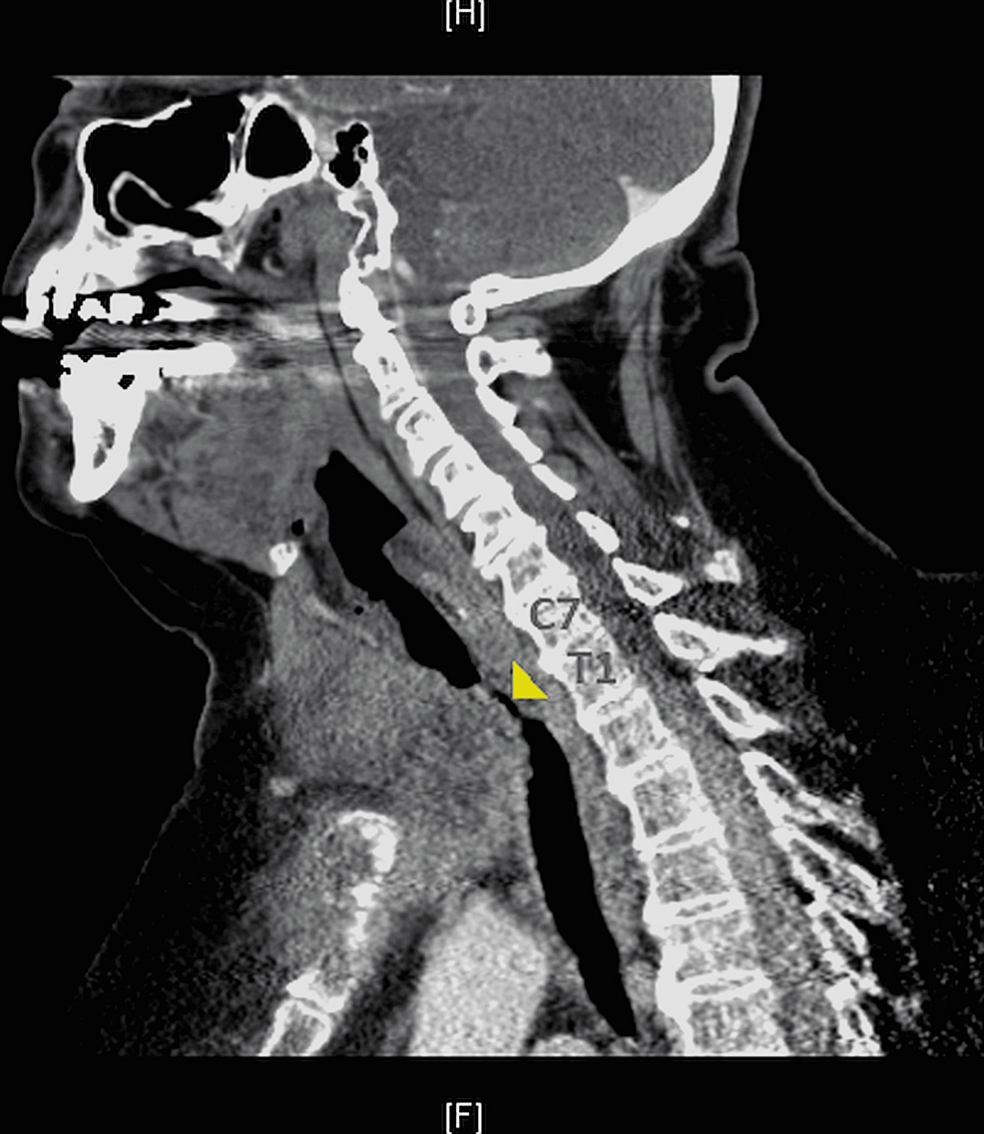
Sagittal CT image of the chest Tracheal stenosis (yellow arrow tip) is visible at the inferior C7 and T1 vertebral levels.
